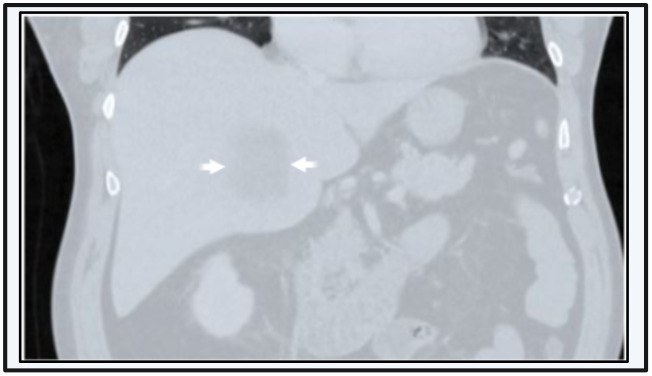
Amebic liver abscess shown on abdominal computed tomography. Returning traveler from an endemic area presenting with an amebic liver abscess seen on computed tomography (CT) imaging (white arrows).
